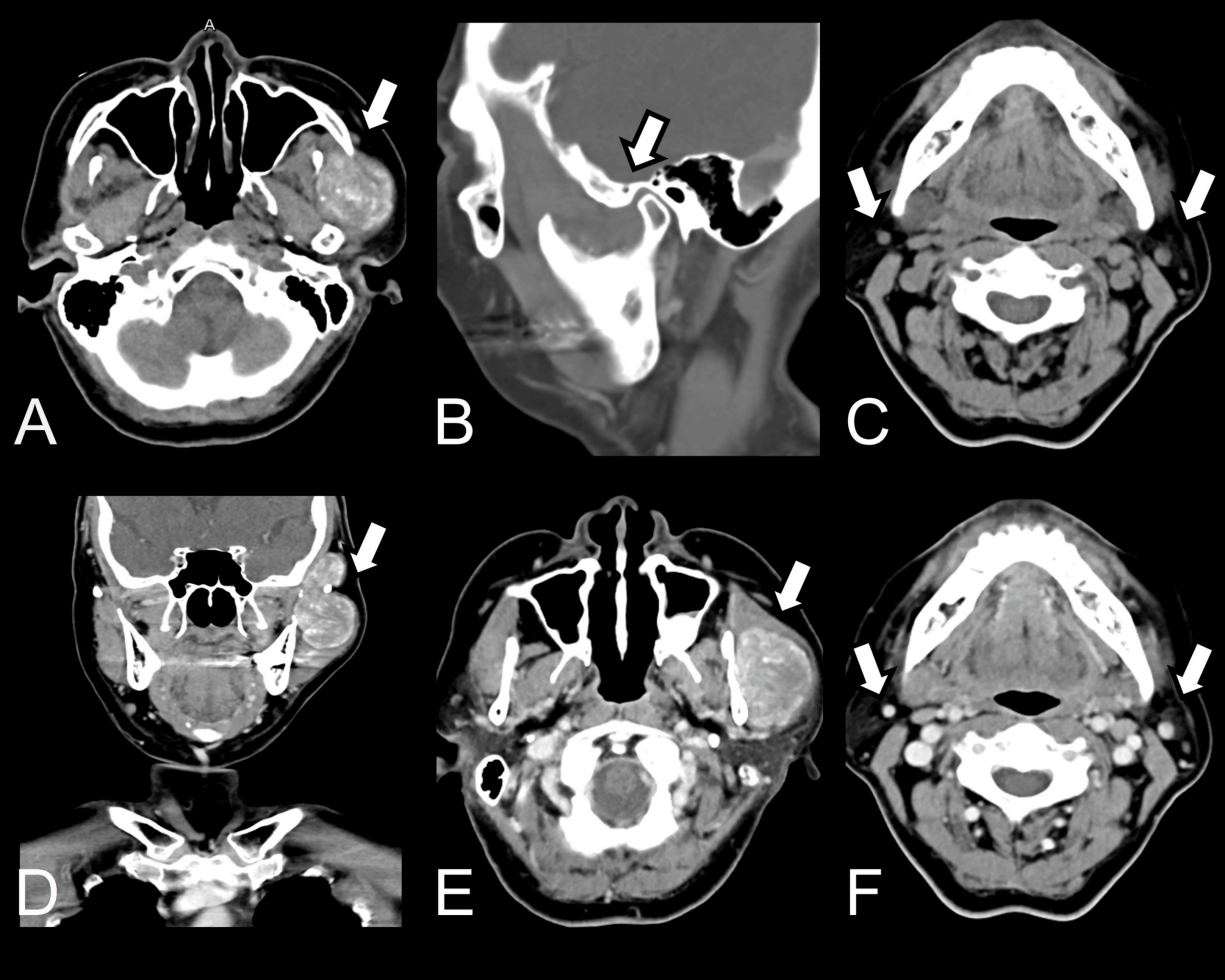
CT scan. (A) Axial view demonstrates a soft tissue mass encasing the left masseteric region and exhibiting internal calcifications. (B) Sagittal view shows no definite erosion of the overlying temporal and mandibular bone. (C and F) Images show non-specific lymph nodes at level IIa. (D and E) Post-contrast images show heterogeneous enhancement. CT: computed tomography
